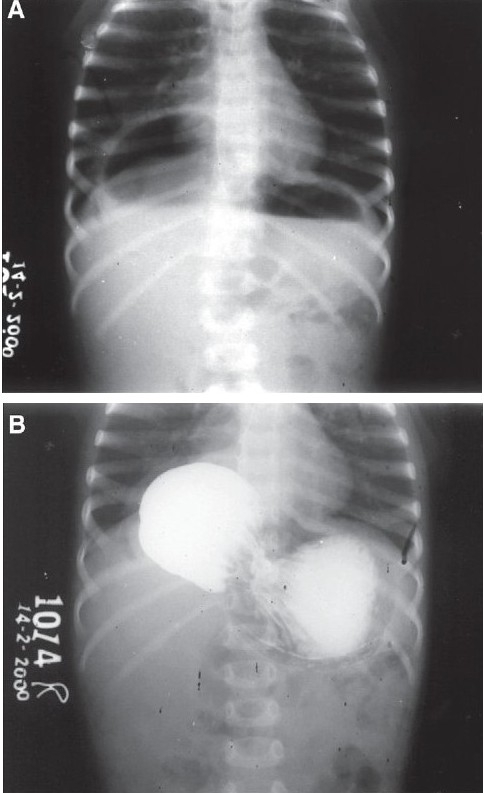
Case 2 (A) Plain X-ray showing right partial eventration (crural eventration). (B) Barium meal picture: mesenteroaxial volvulus
